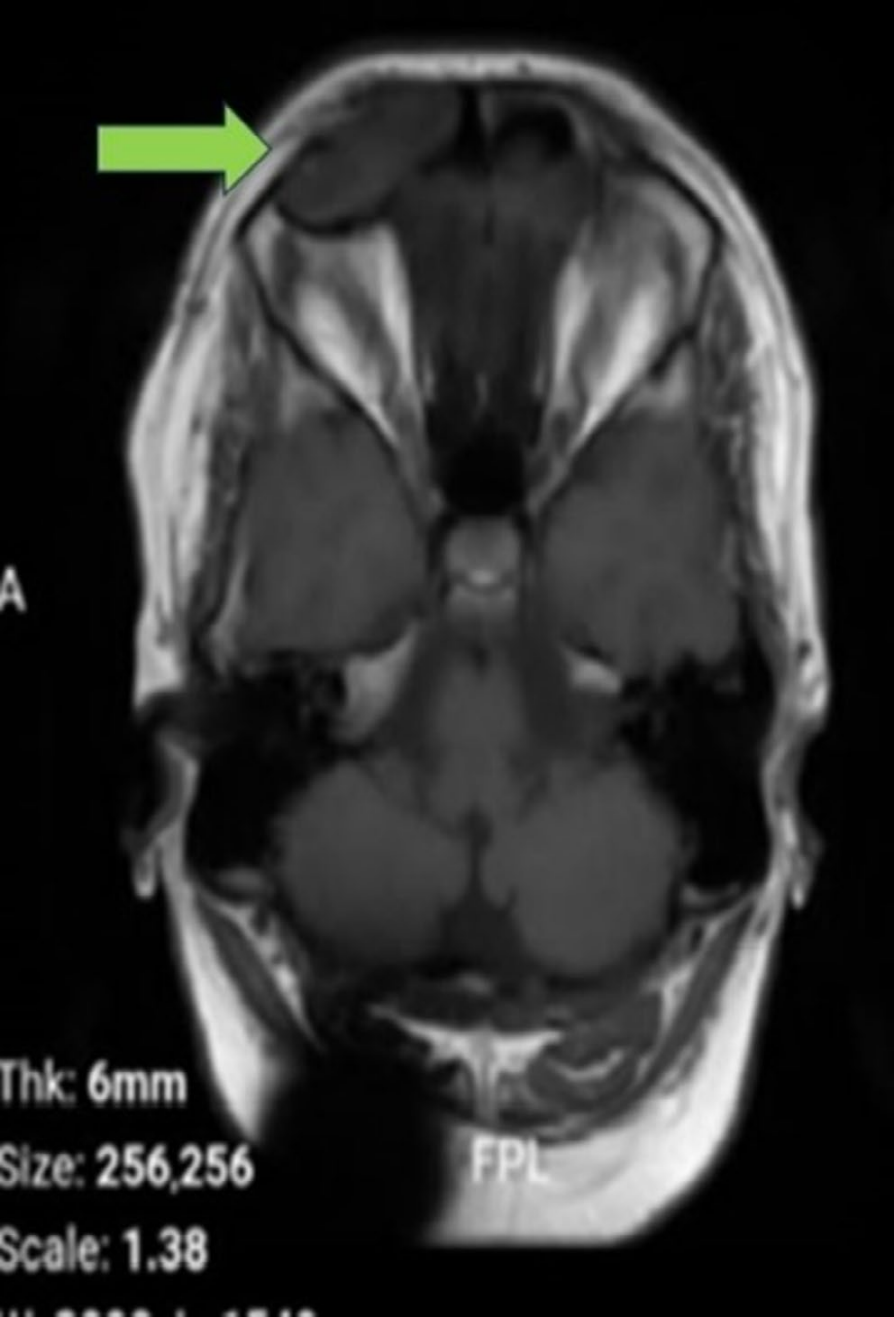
Axial gadolinium‐enhanced T1 MRI confirming non‐enhanced lesion with mass effect on the right orbit causing displacement.
